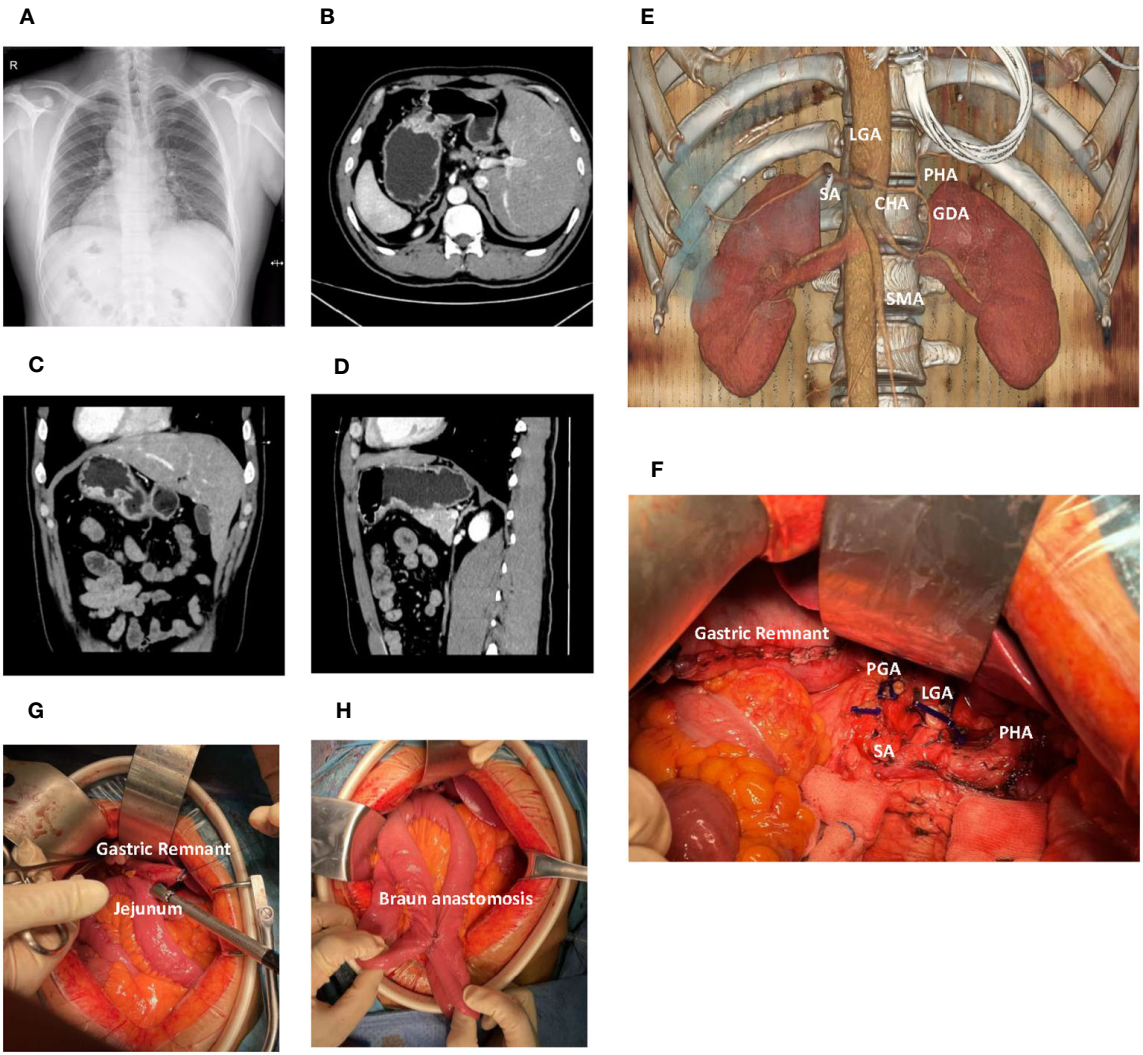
Comprehensive radiological and intraoperative visualization in Situs Inversus Totalis (SIT) accompanied by gastric cancer. (A) Chest X-ray demonstrates dextrocardia and mirror-image arrangement of the thoracic structures. (B) Axial computed tomography (CT) scan reveals a gastric lesion located in the body of the stomach. (C) Coronal CT image shows the gastric lesion in the body of the stomach. (D) Sagittal CT image further delineates the gastric lesion in the stomach body. (E) Three-dimensional vascular reconstruction depicts major vessels such as the celiac trunk and Superior Mesenteric Artery (SMA). (F) The surgical field following distal gastrectomy and D2 lymph node dissection, detailing the altered anatomy and resected regions. (G) The gastrojejunostomy utilizing a linear cutter stapler. (H) The Braun anastomosis. LGA, Left Gastric Artery; PGA, Post Gastric Artery; SA, Splenic Artery; CHA, Common Hepatic Artery; PHA, Proper Hepatic Artery; SMA, Superior Mesenteric Artery.
